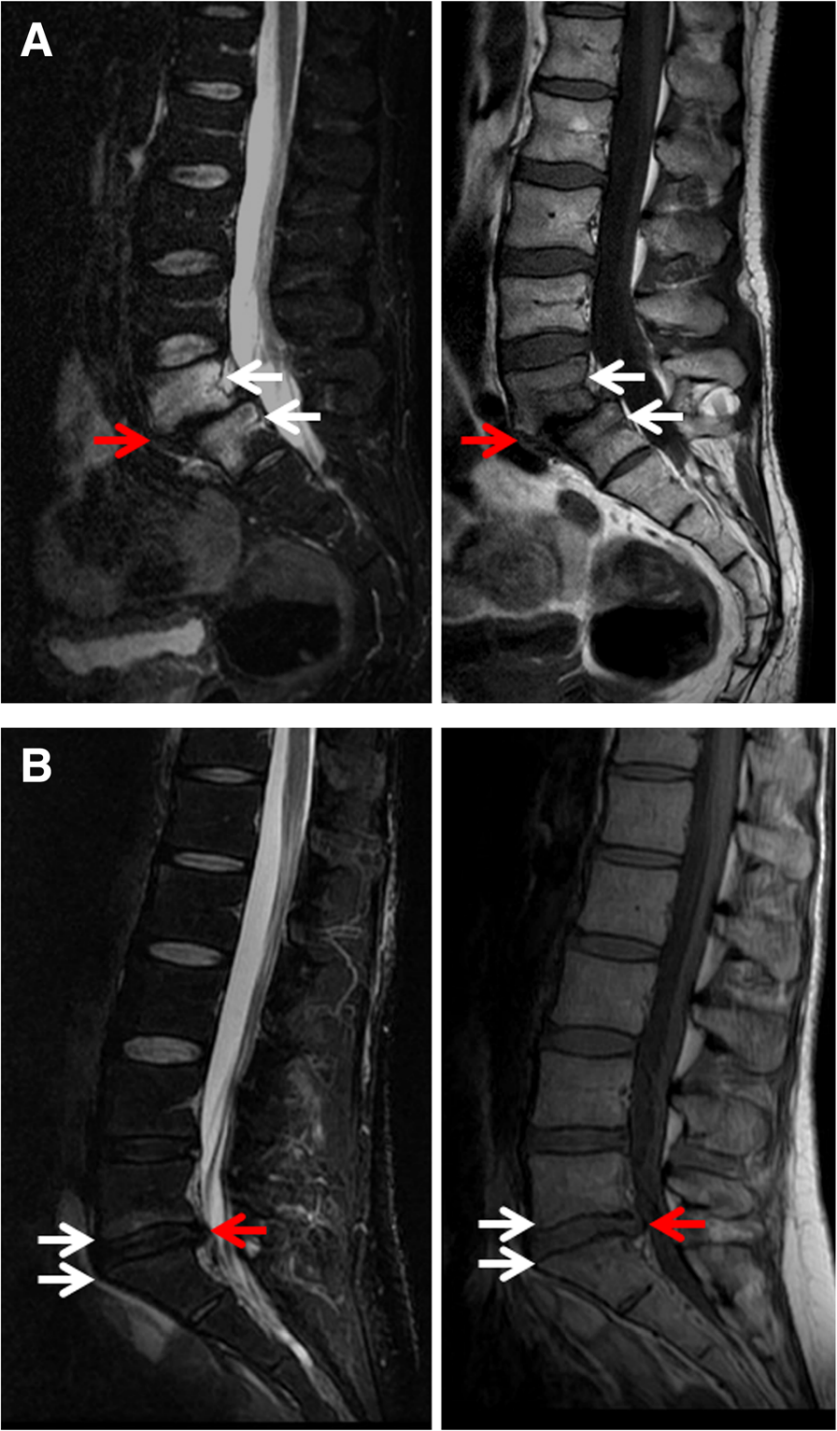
Examples of intervertebral space narrowing >50% intervertebral space narrowing (>50-IVSN) Modic 1 signal changes and ≤50%-IVSN Modic 1 changes on lumbar MRI. a >50%-IVSN Modic 1 changes in L5/S1 in a 52-year-old man. Decrease in intervertebral disc height was >50%, bone edema was present and extended to L5 and S1 vertebral bodies (white arrows) with hyperintense signal in T2-weighted sequence (left panel) and hypointense signal in T1-weighted sequence (right panel), and L5/S1 antelisthesis was observed (red arrow); b ≤50%-IVSN Modic 1 signal changes in L5/S1 in a 35-year-old woman. Decrease in intervertebral disc height was <50%, edema extended to L5 and S1 vertebral endplates (white arrows) with hyperintense signal in T2-weighted sequence (left panel) and hypointense signal in T1-weighted sequence (right panel), and posterior disc protrusion was observed (red arrow). L4/L5 lumbar DDD was also present
